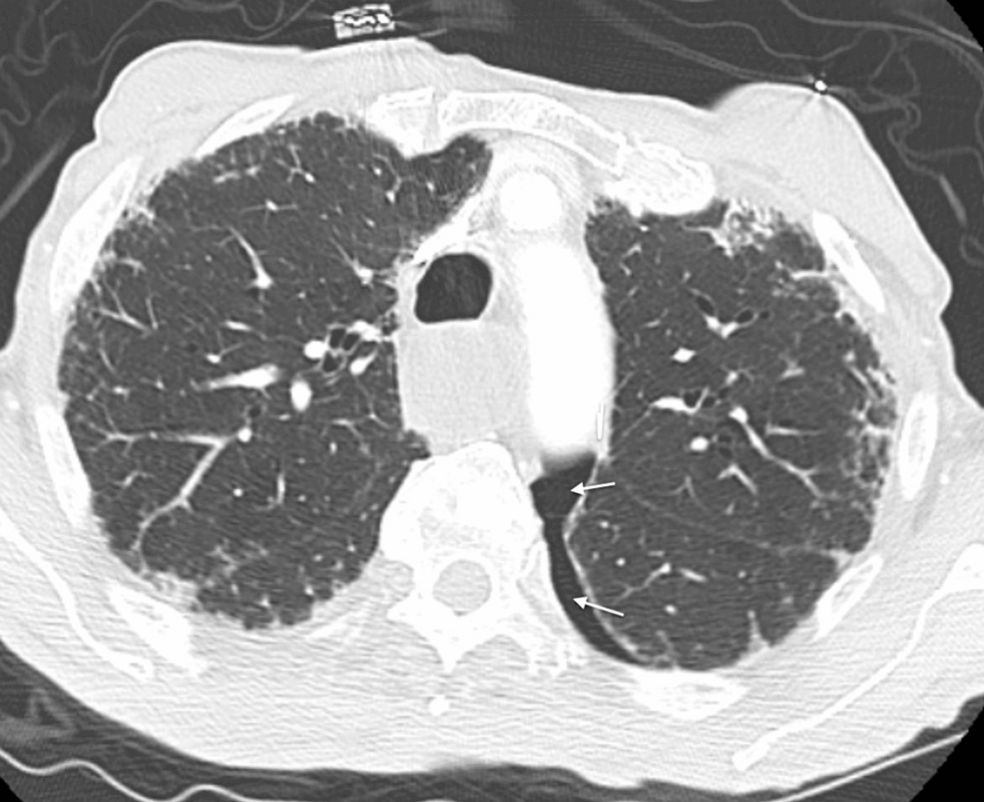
Left pneumothorax "arrows" on admission computed tomography
